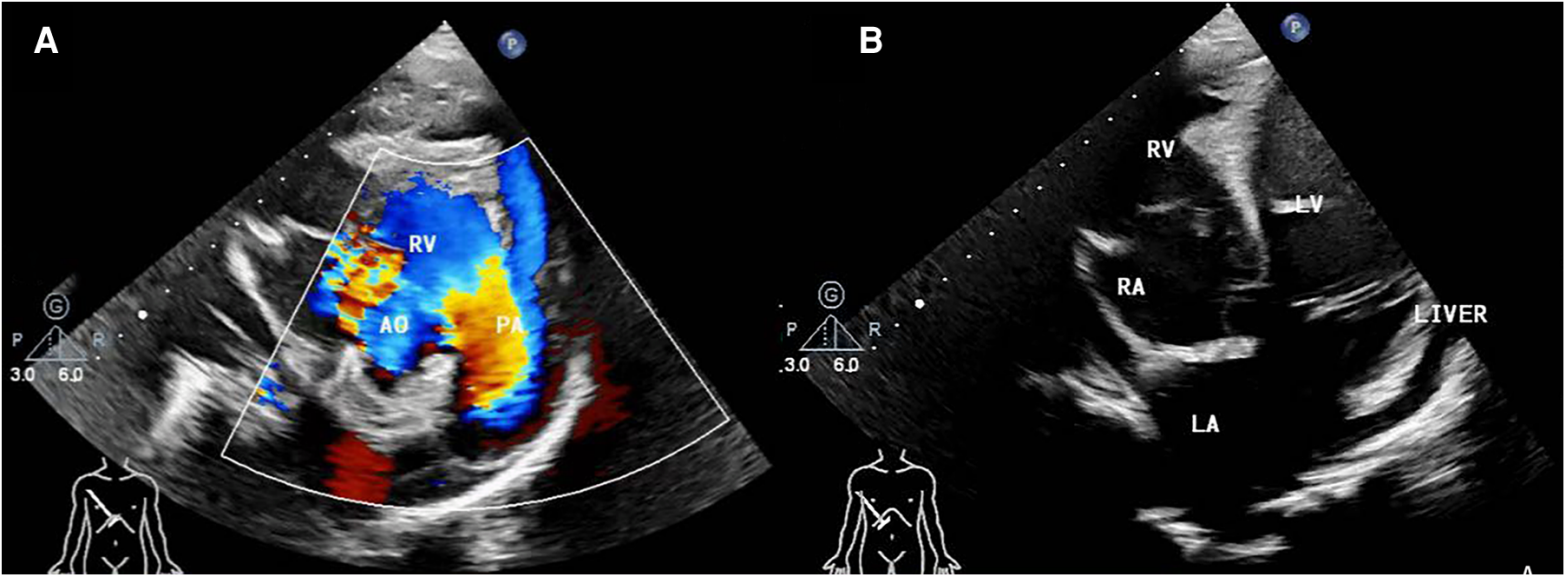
(A) pre-operative transthoracic echocardiogram in right parasternal short axis view, (B) right subcostal arch four-chamber view. AO, aorta; RV, right ventricle; PA, pulmonary artery; RA, right atria; LV, left ventricle; LA, left atria.
